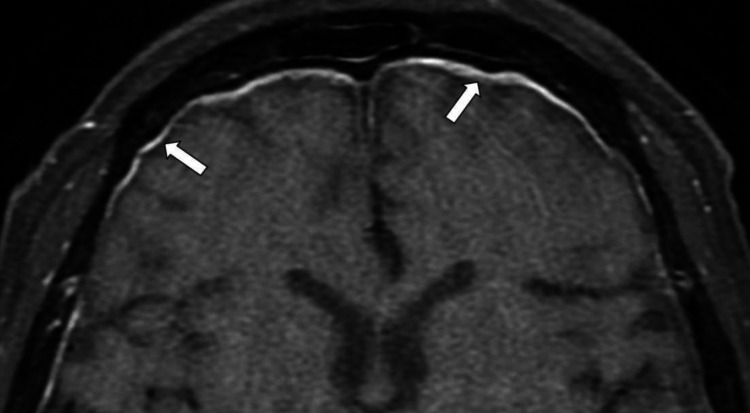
Axial T1-weighted (fat suppression) post-contrasted MRI of the brain. MRI of the brain showed thickened and enhancing pachymeninges (white arrows).
